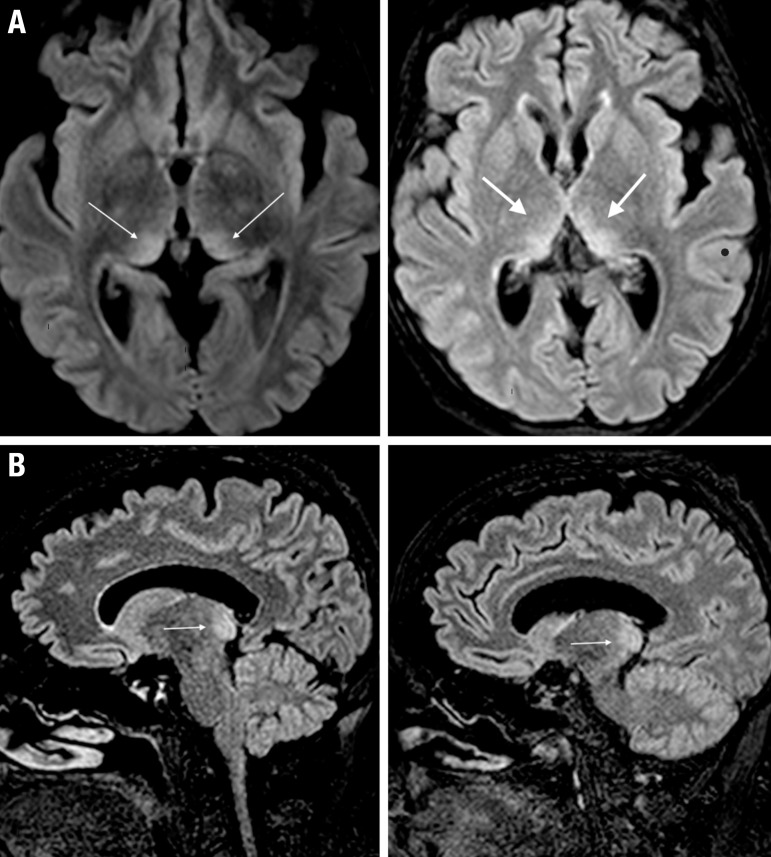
[A] Axial FLAIR-weighted MRI images showing signal
